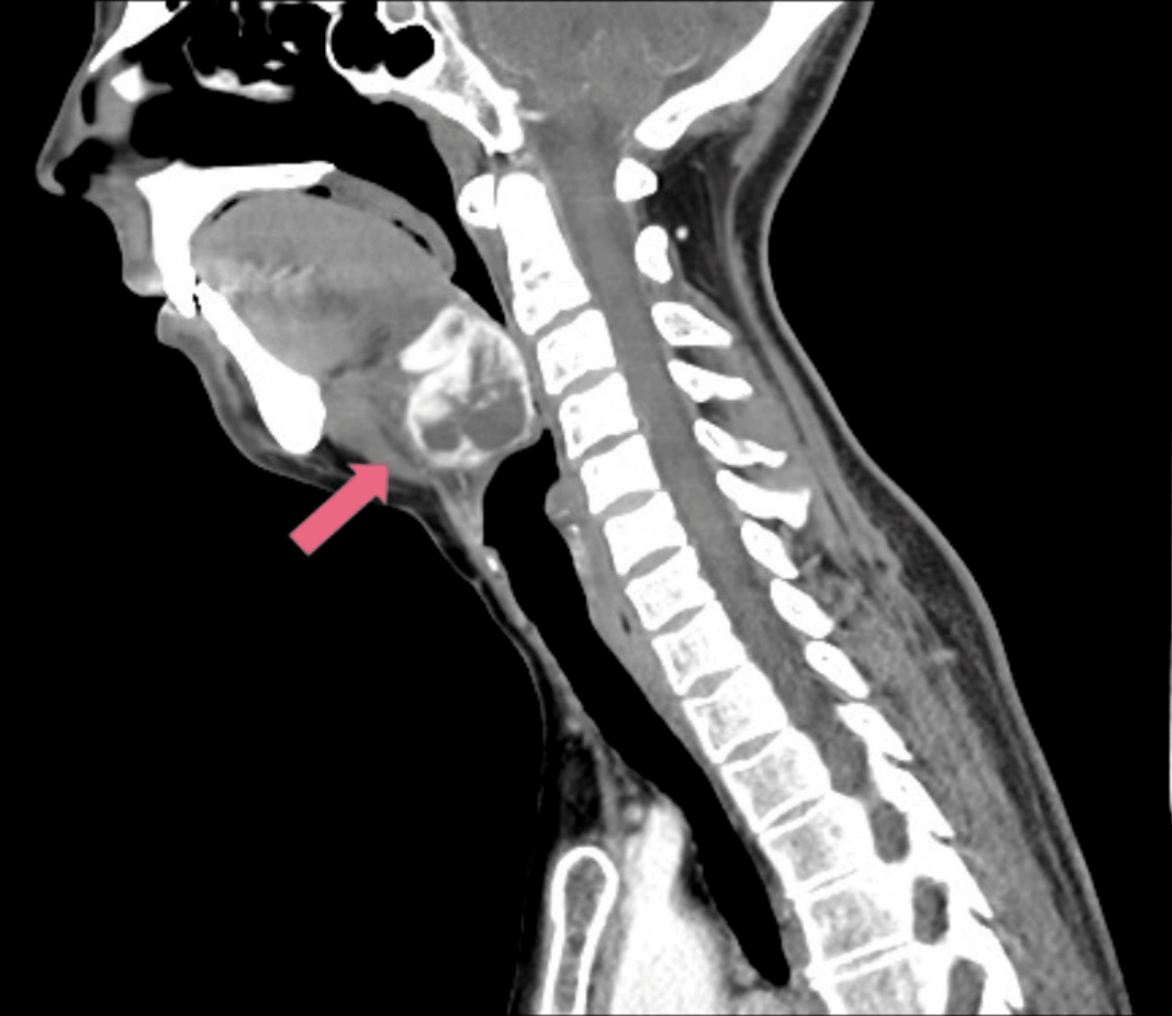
Neck CT scan (sagittal view) Large cystic mass (pink arrow) inferior to the tongue mimicking a thyroglossal duct cyst.
